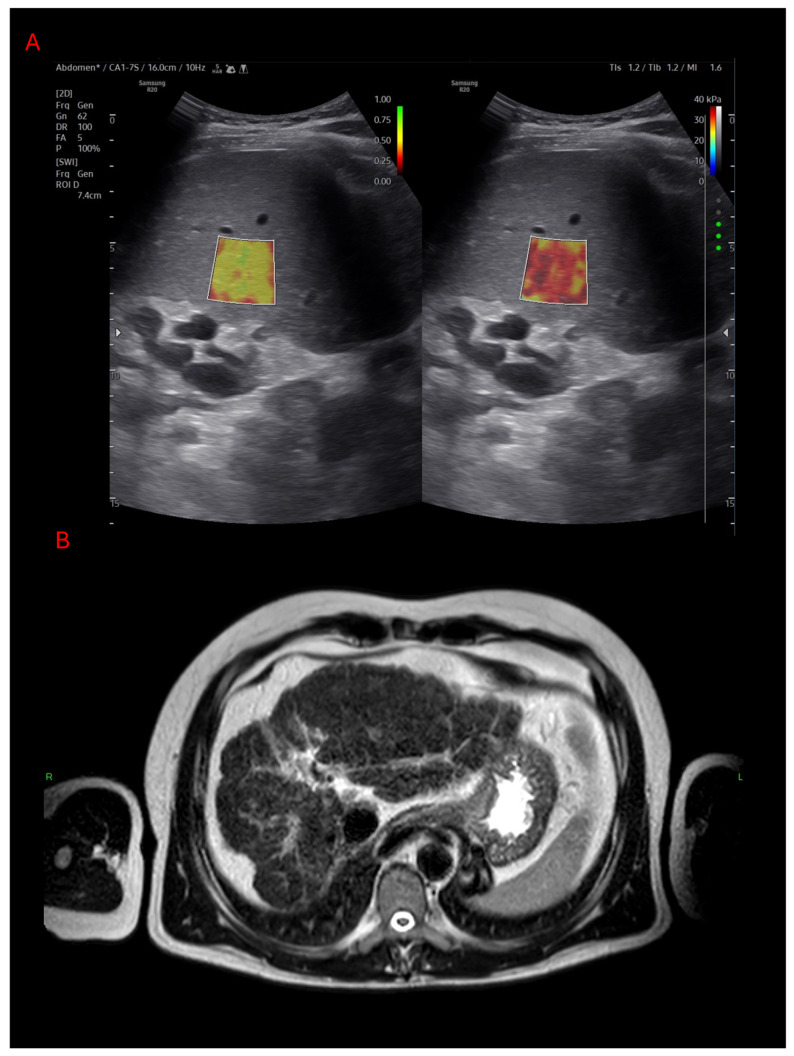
Cirrhosis hepatis in advanced Wilson’s disease. (A): Severe fibrosis is seen on elastography (B): Advanced cirrhosis with ascites seen on T2-weighted MRI scan.
